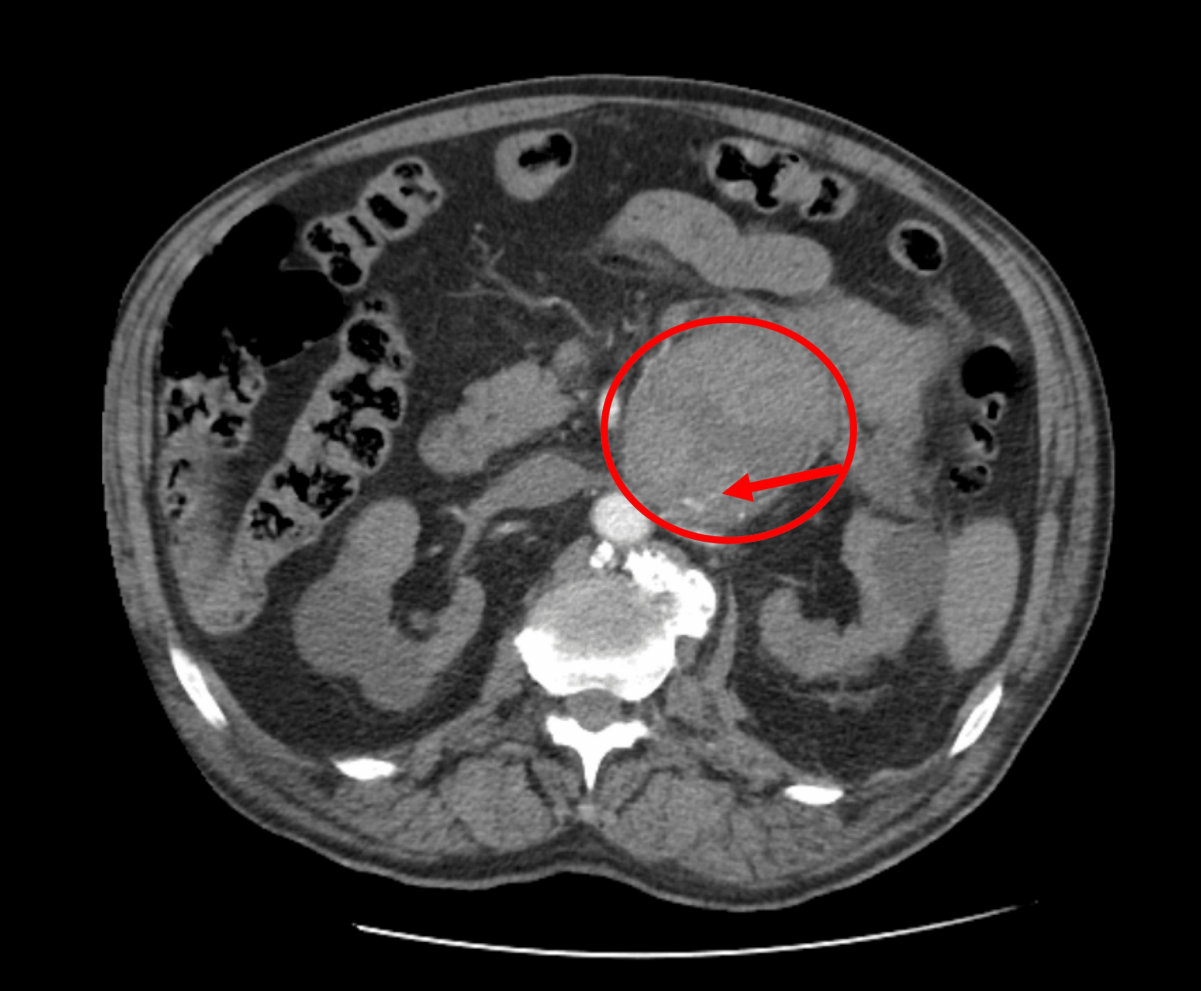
Abdominal computed tomography at admission, in the arterial phase, showing vascular blush suggestive of active bleeding (arrow)
